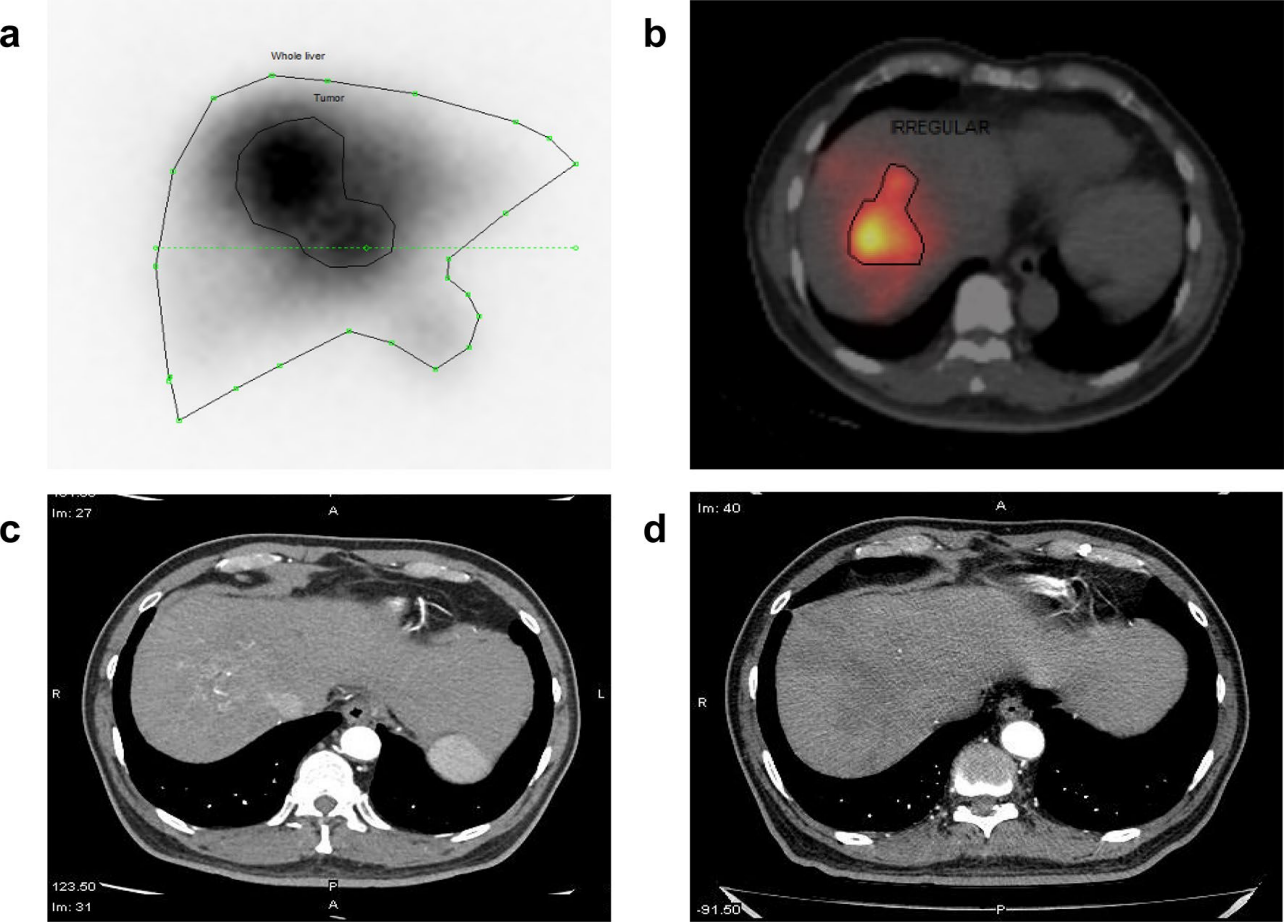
Images of a representative case. A 70-year-old male diagnosed with advanced stage of HCC, underwent ^99m^Tc-MAA planar scan (a) and SPECT/CT (b). Planar image showed only heterogeneous tumor uptake in the right liver, whereas SPECT/CT depicted better tumor margin by delineating viable tumor with excluding necrotic part (b). In comparison with CT before treatment (c), complete treatment response was seen on follow-up CT after 2 months (d).
